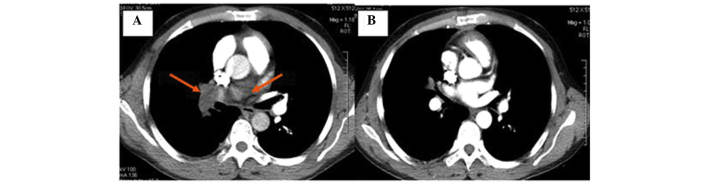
A representative computed tomography image (A) prior to surgery (10/27/2012), and (B) following surgery (11/30/2012). Arrows indicate the location of the pulmonary artery leiomyosarcoma tumor prior to surgery.
